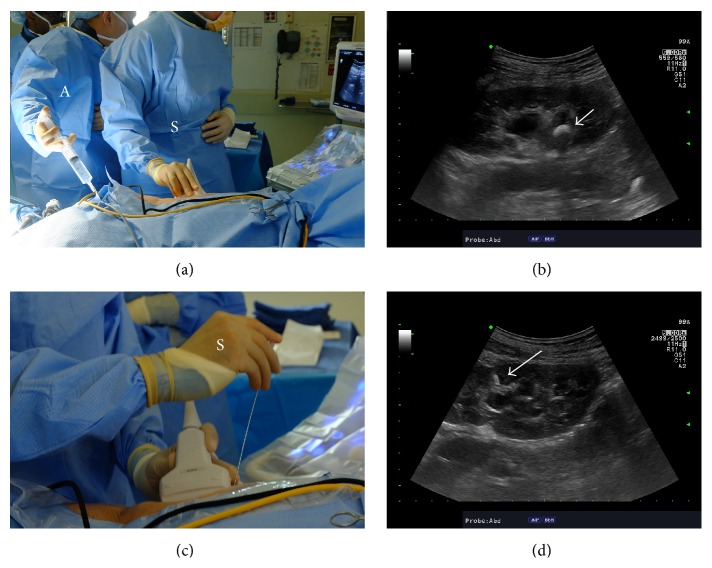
Establishing renal access using ultrasound guidance. (a) The operative surgeon (S) holds the ultrasound probe and the assistant (A) holds a syringe attached to ureteral catheter for normal saline infusion if needed. (b) Ultrasonographic image of the kidney along its longitudinal axis demonstrating the stone in the renal pelvis (white arrow) within a mildly hydronephrotic collecting system. (c) During the needle insertion, the operative surgeon (S) holds both the ultrasound probe and the needle to perform the puncture. For hand positioning, the nondominant hand holds the ultrasound probe while the dominant hand holds the needle. (d) The needle can be visualized (white arrow) entering the collecting system through upper pole calyx in this case.
